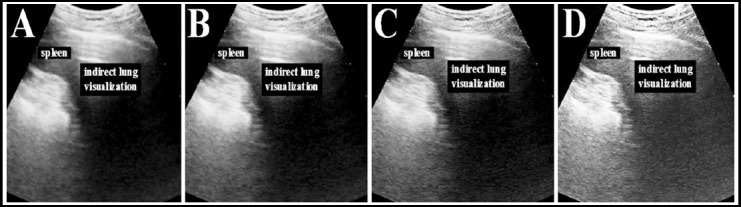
Comparison of the reconstruction effect of three algorithms on a female neonate’s lung ultrasound image (age 8 days, weight 2.85kg). (A, B, and C had the same indications with Fig.3).
